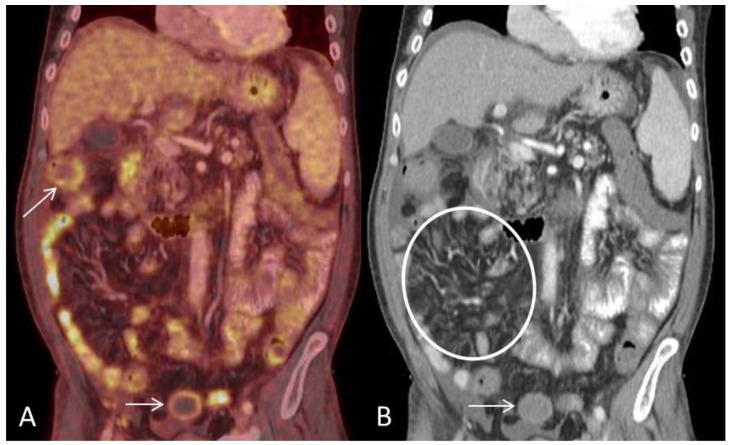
(A) Coronal PET and CT fused image demonstrates multifocal segmental pathological FDG-uptake in small-and large bowel loops. Circumferential mural pathological FDG-uptake in the large bowel (arrows). (B) Coronal CT shows engorgement of the vasa recta (circle) and mural enhancement of the large bowel (arrow). Ascites is present on the surface of the liver. Engorgement of the vasa recta is due to increased blood-flow in small arteries of inflamed bowel segments and has been reported as the most consistent extraintestinal finding in acute GIT-GVHD [2].
